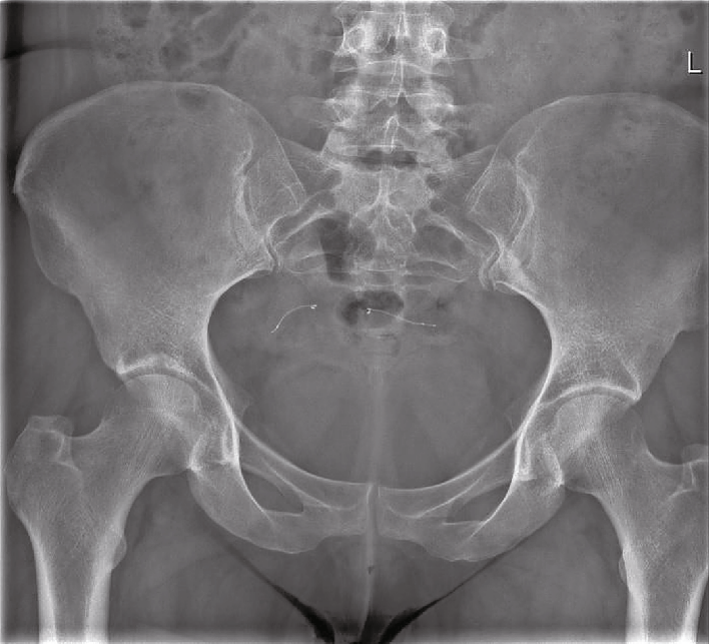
Flat panel abdominal X-ray (anterior posterior view) showing both implants (ESS 205) with symmetrical deployment in the pelvic area and the proximal markers are 25 mm apart.
